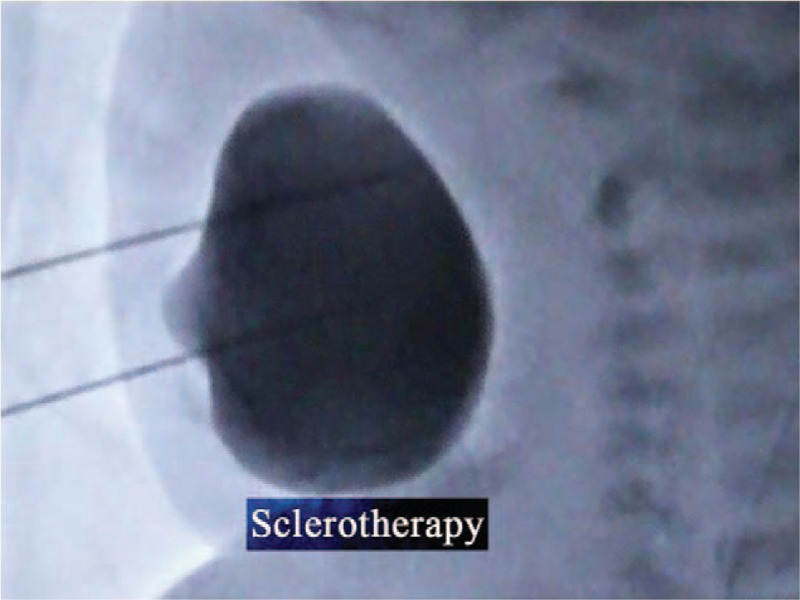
The radiography image. The radiography image showed that mixed infusion was deposited in the lesion.
